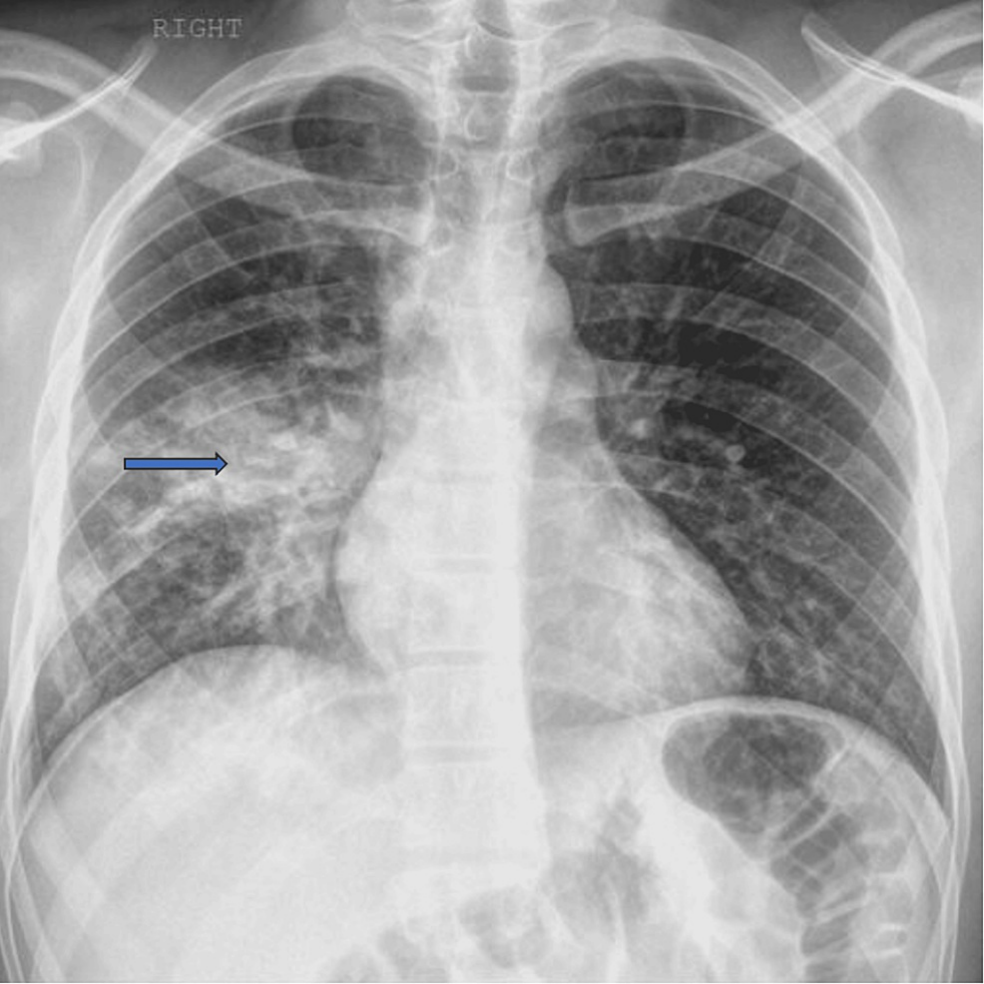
X-ray of the chest during hospital admission The blue arrow shows consolidation of the right lung lobe. The presence of other acute cardiopulmonary abnormality is ruled out.
